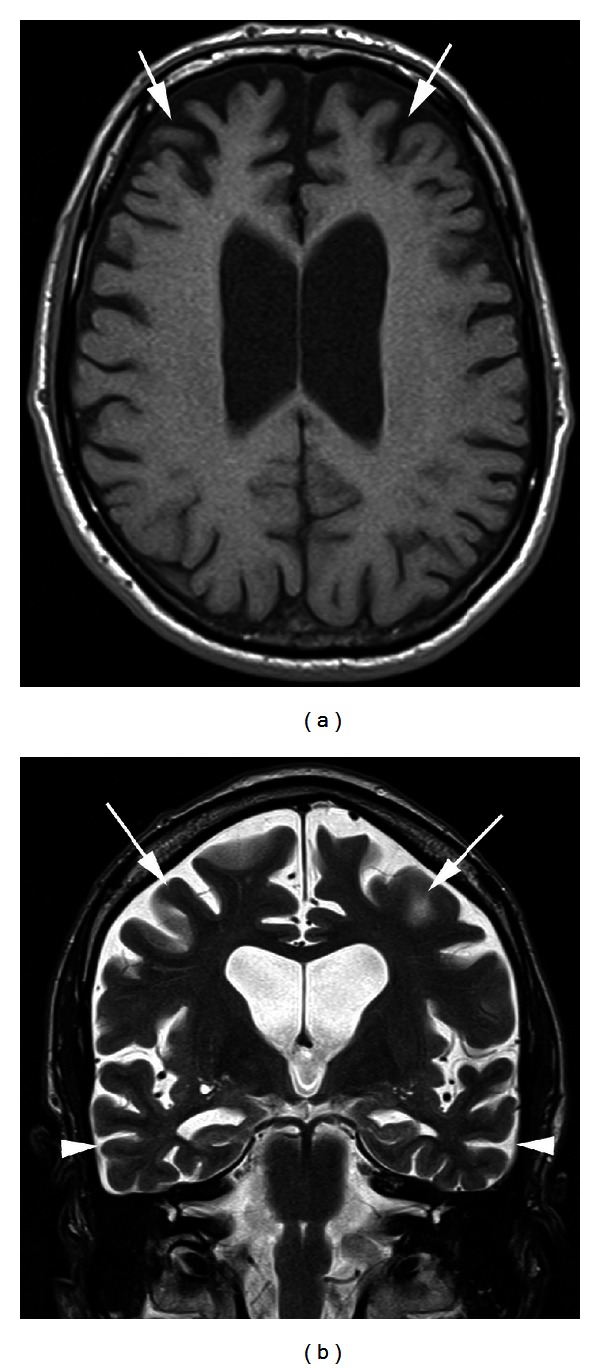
(a) An axial T1-weighted MR image of the brain demonstrating severe bilateral frontal lobe atrophy (arrows) compared to the parietal lobes. (b) A coronal T2-weighted MR image demonstrating severe bilateral frontal lobe atrophy (arrows) as well as bilateral temporal lobe atrophy (arrowheads).
